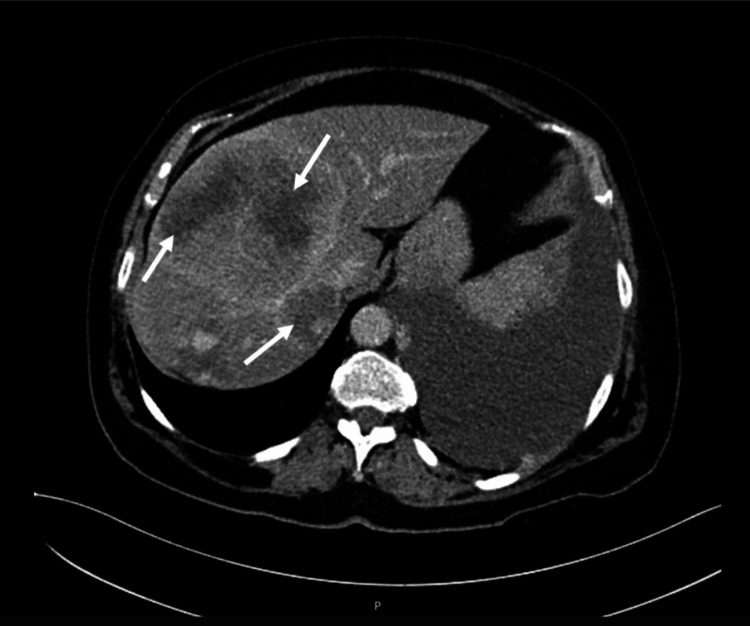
Abdominal CT scan with liver nodules suggestive of metastases.
